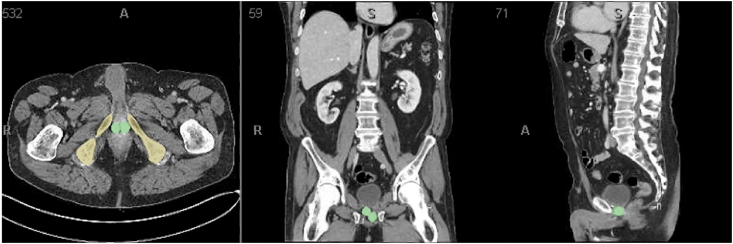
CT pelvis demonstrating optimal balloon position (green) adjacent to the pubic bone (yellow) in a different patient. (For interpretation of the references to colour in this figure legend, the reader is referred to the Web version of this article.)
